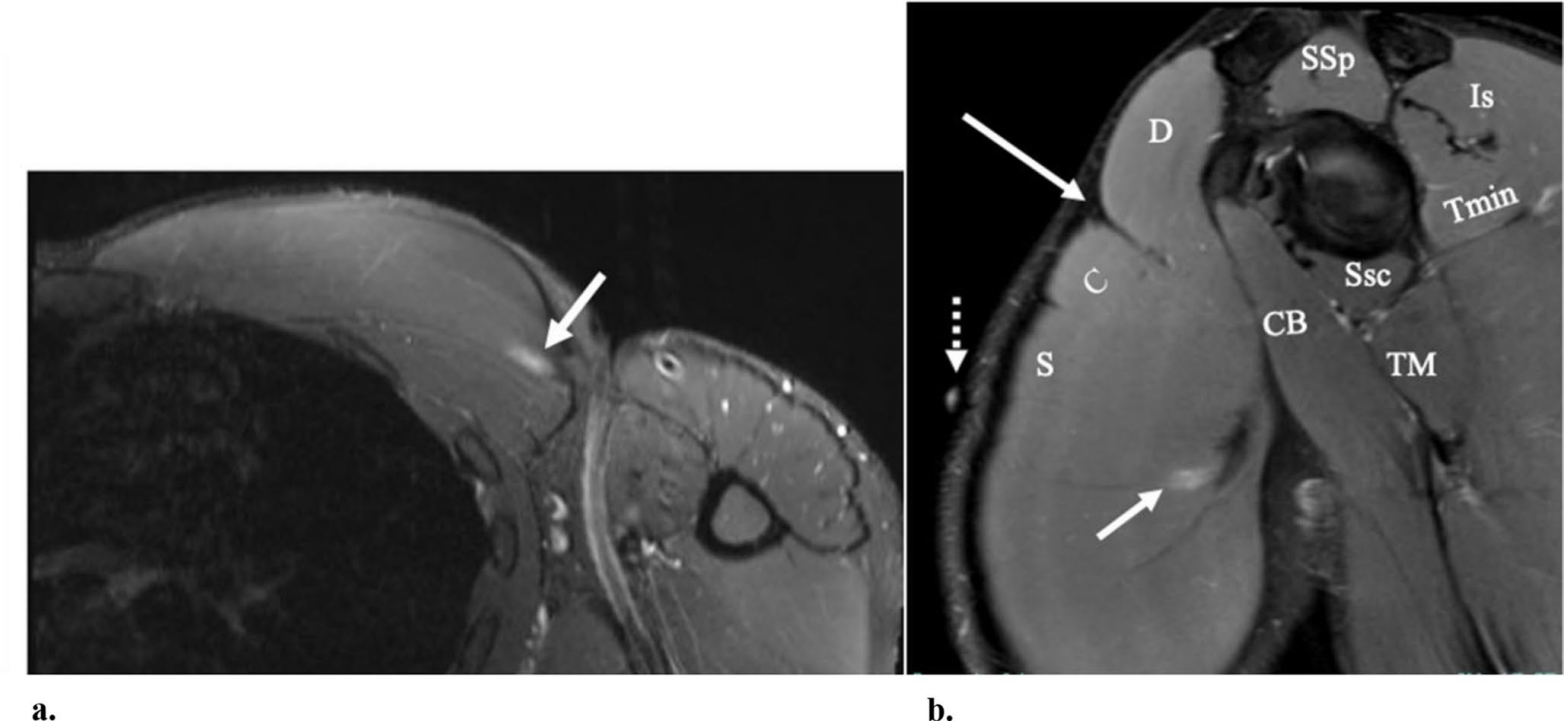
24-year-old-male with pain at the site of the external marker (dashed arrow in b). (a) Axial STIR MR image shows edema-like signal at the myotendinous junction of the pectoralis major (arrow), related to partial width tear at this site. (b) Sagittal proton-density with fat-suppression also shows the partial tear at the myotendinous junction of the sternal head (short arrow). Note the deltopectoral groove (long arrow) between the deltoid muscle (D) and the clavicular head (C) of the pectoralis major muscle. The sternal head (S) of the pectoralis major muscle is shown. The supraspinatus muscle (SSp), infraspinatus muscle (Is), teres minor muscle (Tmin), teres major muscle (TM), subscapularis muscle (Ssc), and the corachobrachialis muscle (CB) are also denoted
